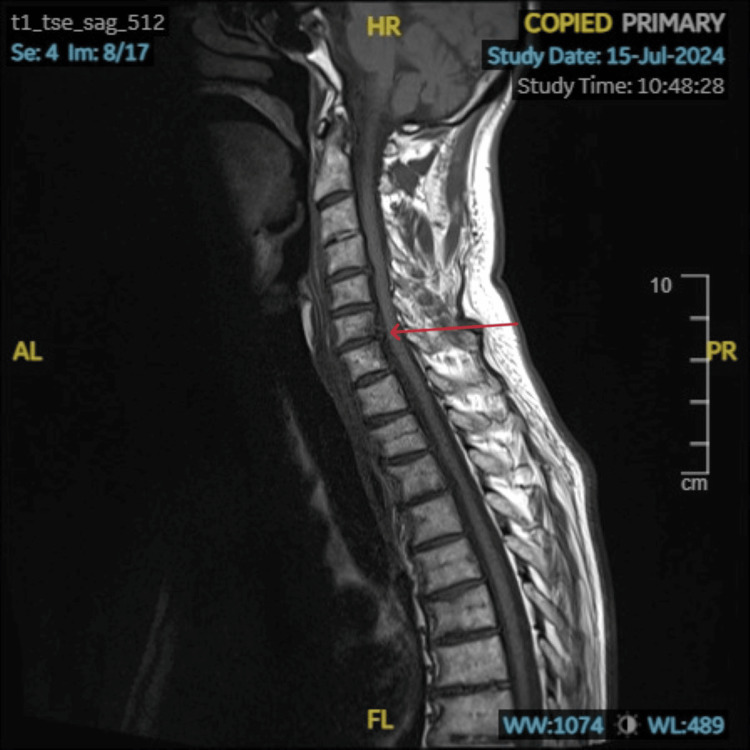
MRI scan of the spine Fracture with surrounding marrow edema/contusion of the left superior articular process at the C7 level and an undisplaced fracture involving the left transverse process at the C7. There is minimal edema involving either aspect of the C6-C7 level intervertebral disc and prevertebral edema in the cervical spine. This is marked with a red arrow.
